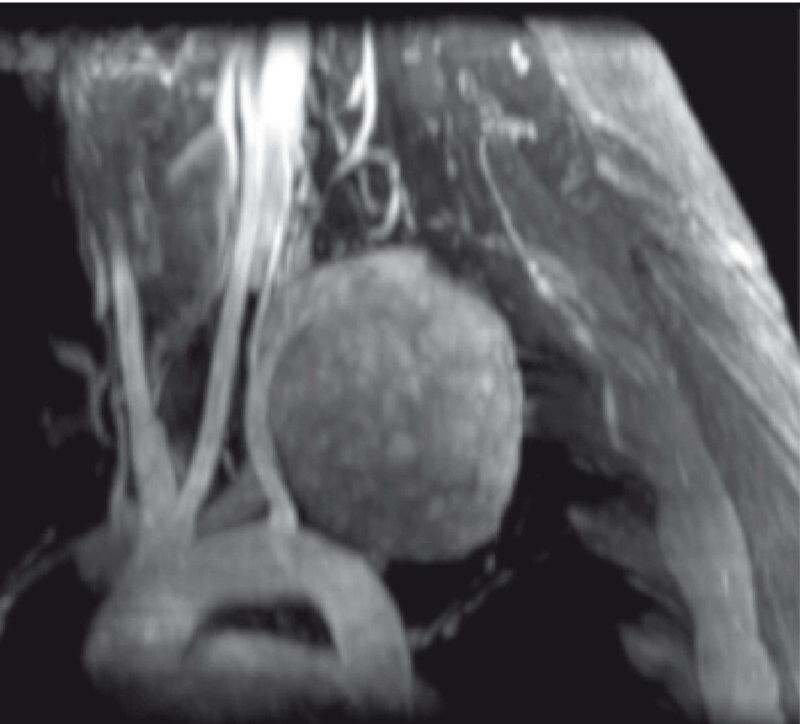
Computed tomography 3 D reconstruction with a nodular mass of approximately 7 cm in diameter in the left apical pleura with compression of the subclavian artery.
